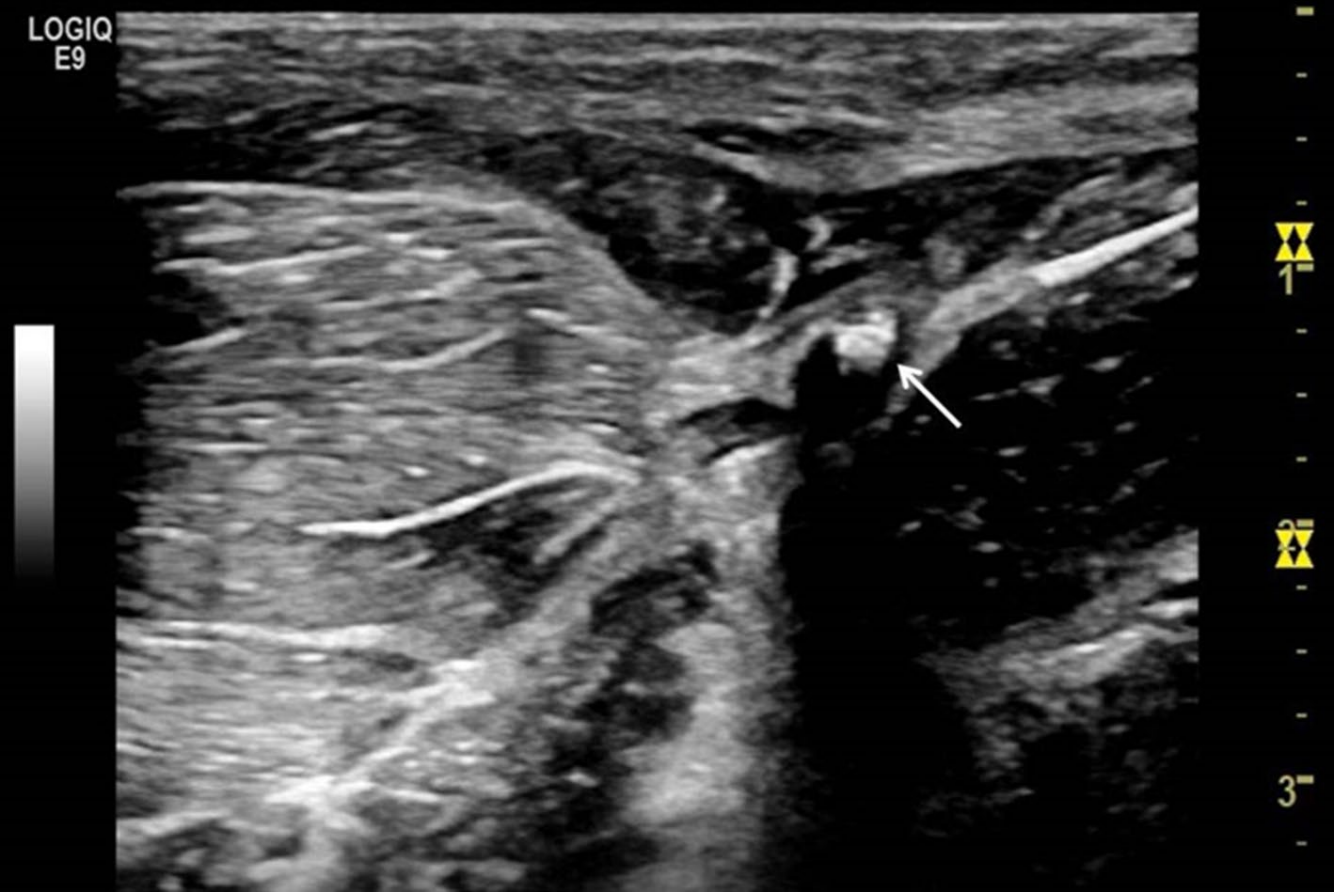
Ultrasonography of the femoral artery confirms ANGIO-SEAL^®^ VIP Vascular Closure Device placement.
